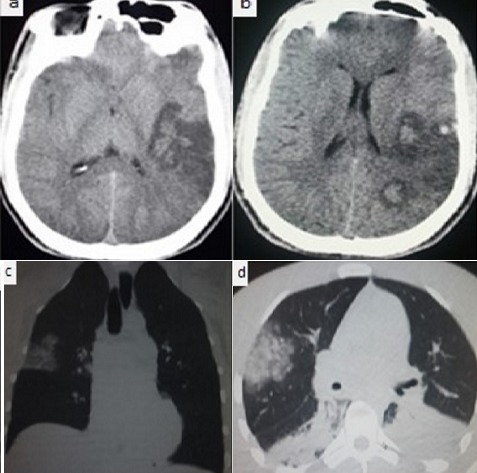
A computed tomography: (a, b) axial images of brain: show area of stroke in the left middle artery territory with hemorrhagic infarction; (c,d) chest coronal and axial images, in lung window: demonstrate an area of intra alveolar bleeding, involving the superior right pulmonary lobe and both the Fowler segment
